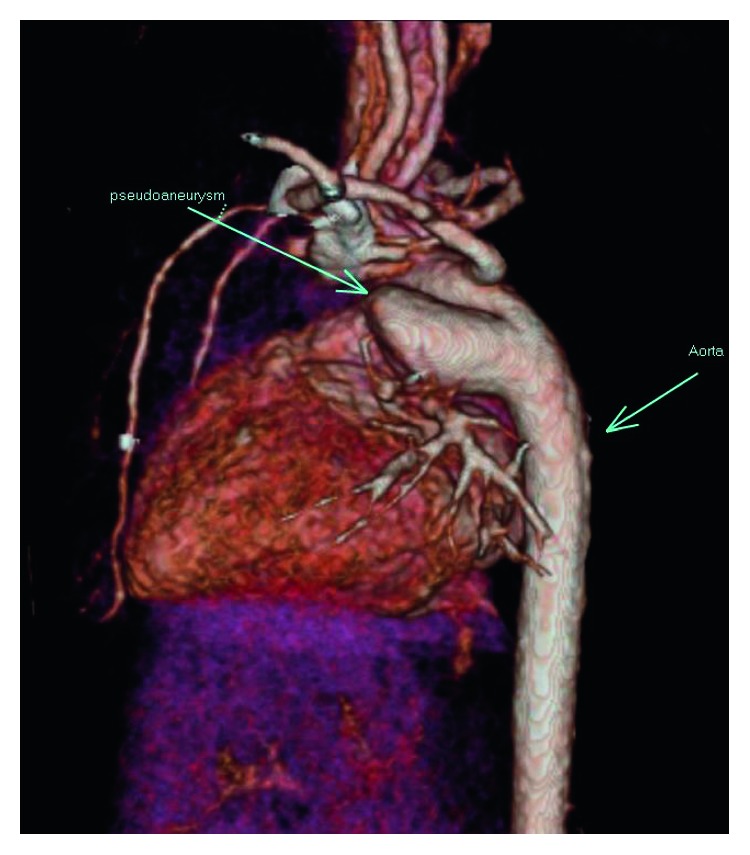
Three-dimensional volume rendering of the thoracic CT-A revealing pseudoaneurysm and relationship with aorta and left pulmonary artery.
